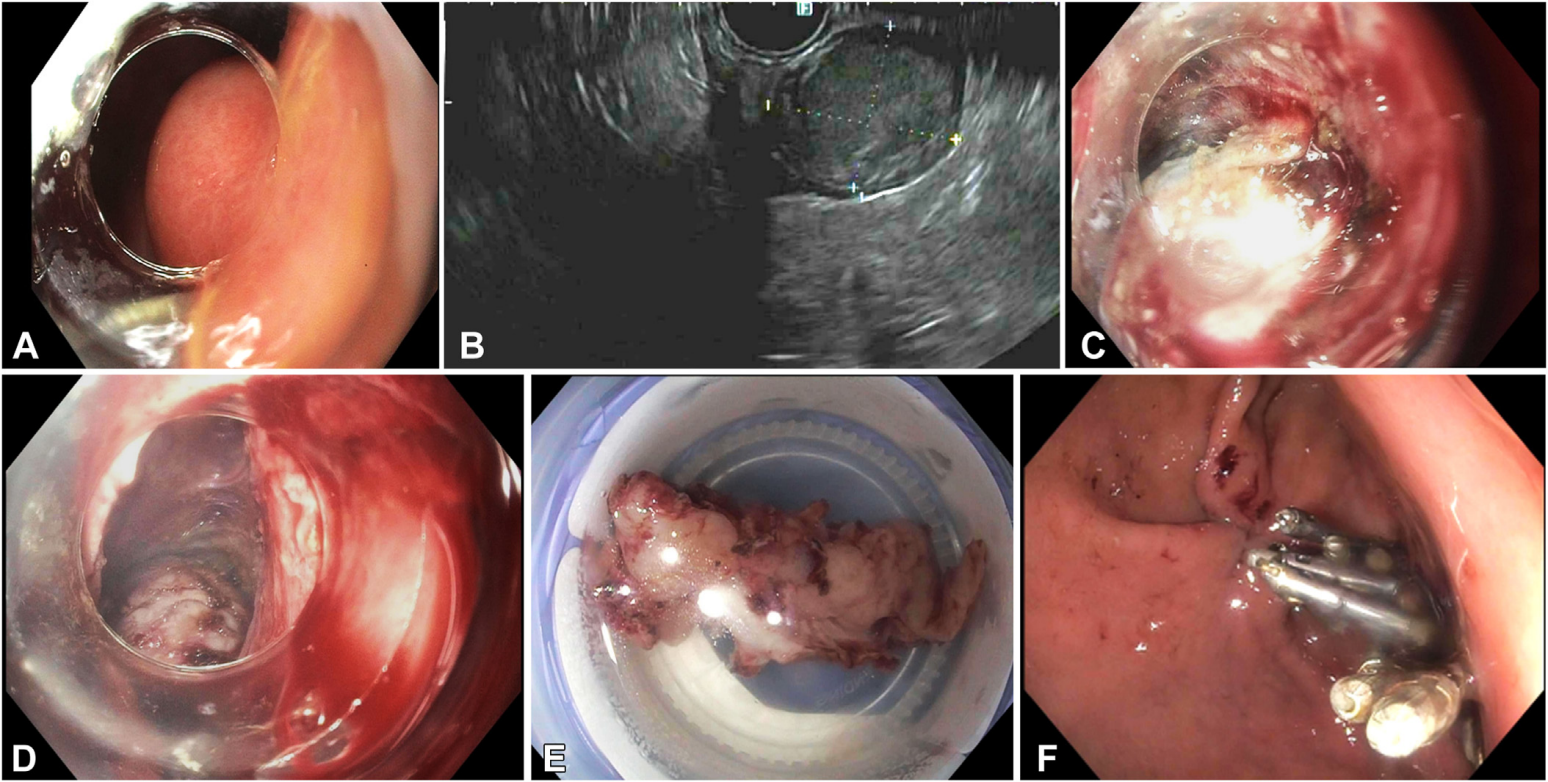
Patient 4. A, Endoscopic view of the gastric subepithelial lesion. B, Endosonographic view of the gastric SEL. C, Endoscopic view of the SEL after submucosal tunneling. D, Endoscopic view of the SEL after dissecting its attachments to the muscularis propria. E, The extracted gastric SEL measuring 5 cm. F, View after closing the tunnel entry with endoscopic suturing and clips. *SEL*, Subepithelial lesion.
